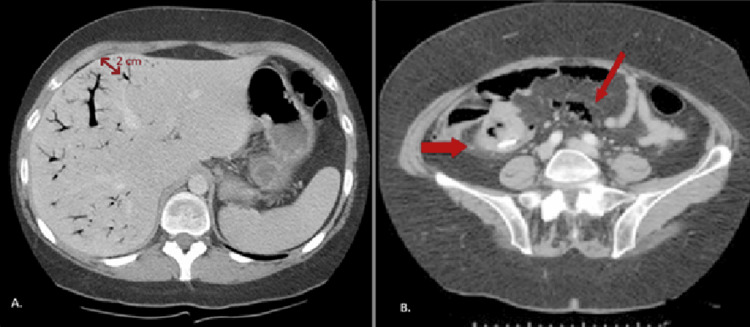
CT findings demonstrating portal venous gas and mesenteric air Initial CT of the abdomen and pelvis without contrast. (A) Axial image demonstrating extensive portal venous gas (arrows) with branching lucencies extending to within 2 cm of the liver capsule [3]. (B) Axial image showing a linear branching pattern of gas within the mesenteric veins (red arrow) with associated mild mesenteric stranding (red thick arrow) [7].
